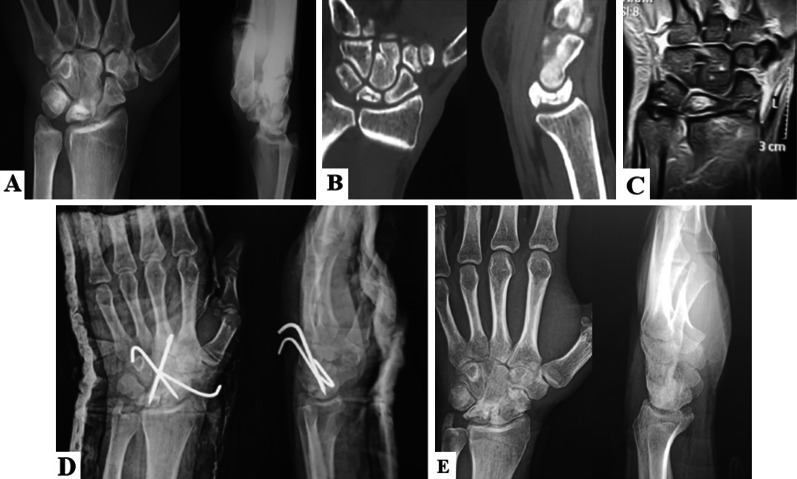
A case of a male patient, 33 years old, with grade IIIA Kienböck’s disease treated with partial lunate excision and scapho-luno-capitate fusion. A Preoperative X-rays, anteroposterior and lateral views, showing avascular necrosis and collapse of the lunate. B Preoperative coronal and sagittal CT scans showing collapse of the lunate. C Preoperative MRI showing intact proximal articular cartilage of the lunate. D Immediate postoperative X-rays, anteroposterior and lateral views. E One-year follow-up X-rays, anteroposterior and lateral views, showing complete scapho-luno-capitate fusion
